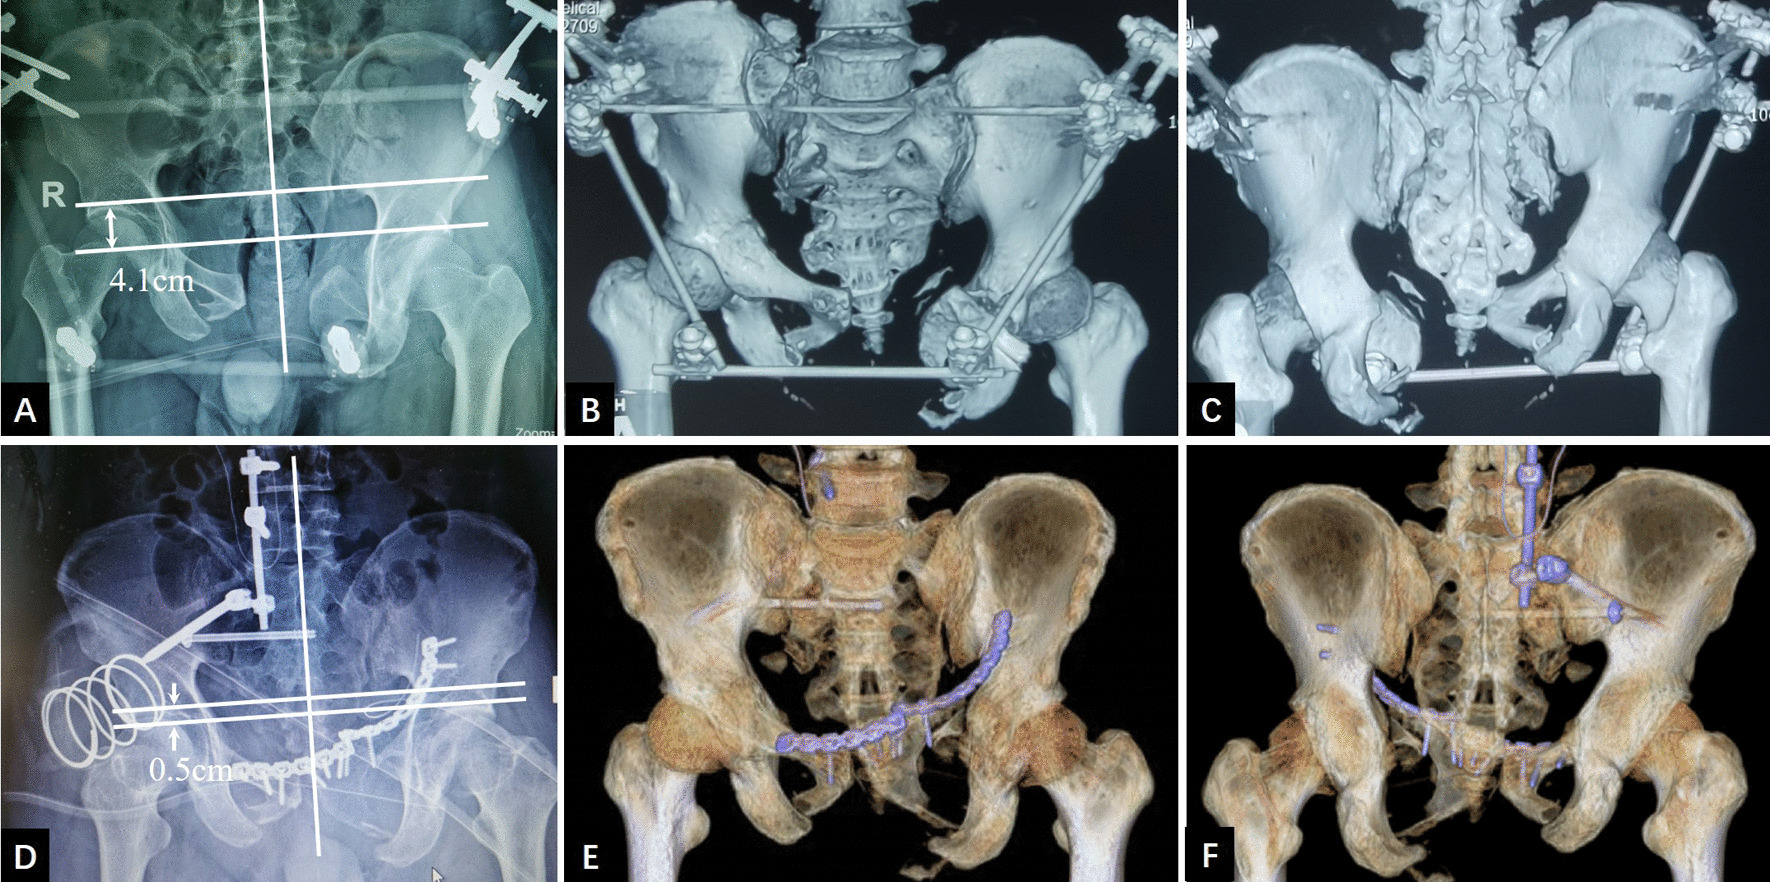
A 32-year-old male patient treated with the prone–supine sequence. a Preoperative AP X-ray showing significant vertical displacement (4.1 cm) of the right hemipelvis. b, c Three-dimensional CT showing separation of the anterior pelvic ring at the pubic symphysis and malunion of the posterior pelvic ring. d–f Postoperative AP X-ray and 3D-CT showing that a residual vertical displacement of 0.5 cm
